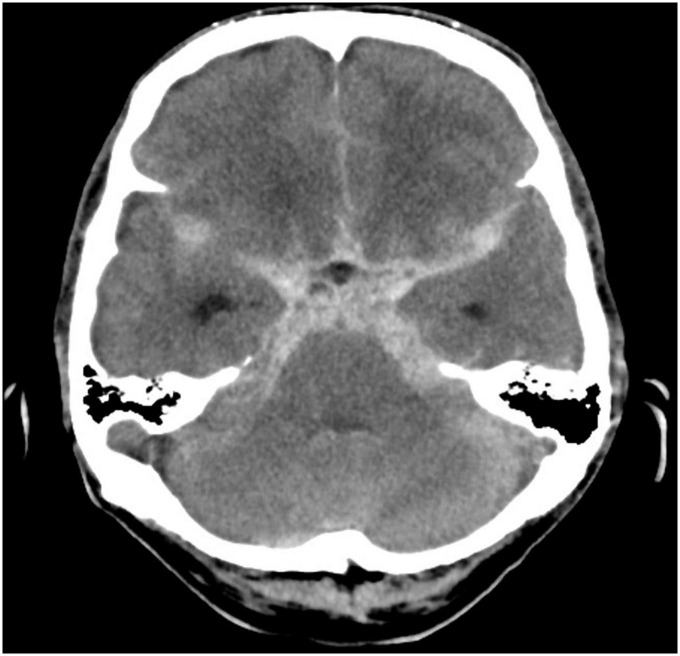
Computed tomography brain showing extensive acute subarachnoid hemorrhage.
